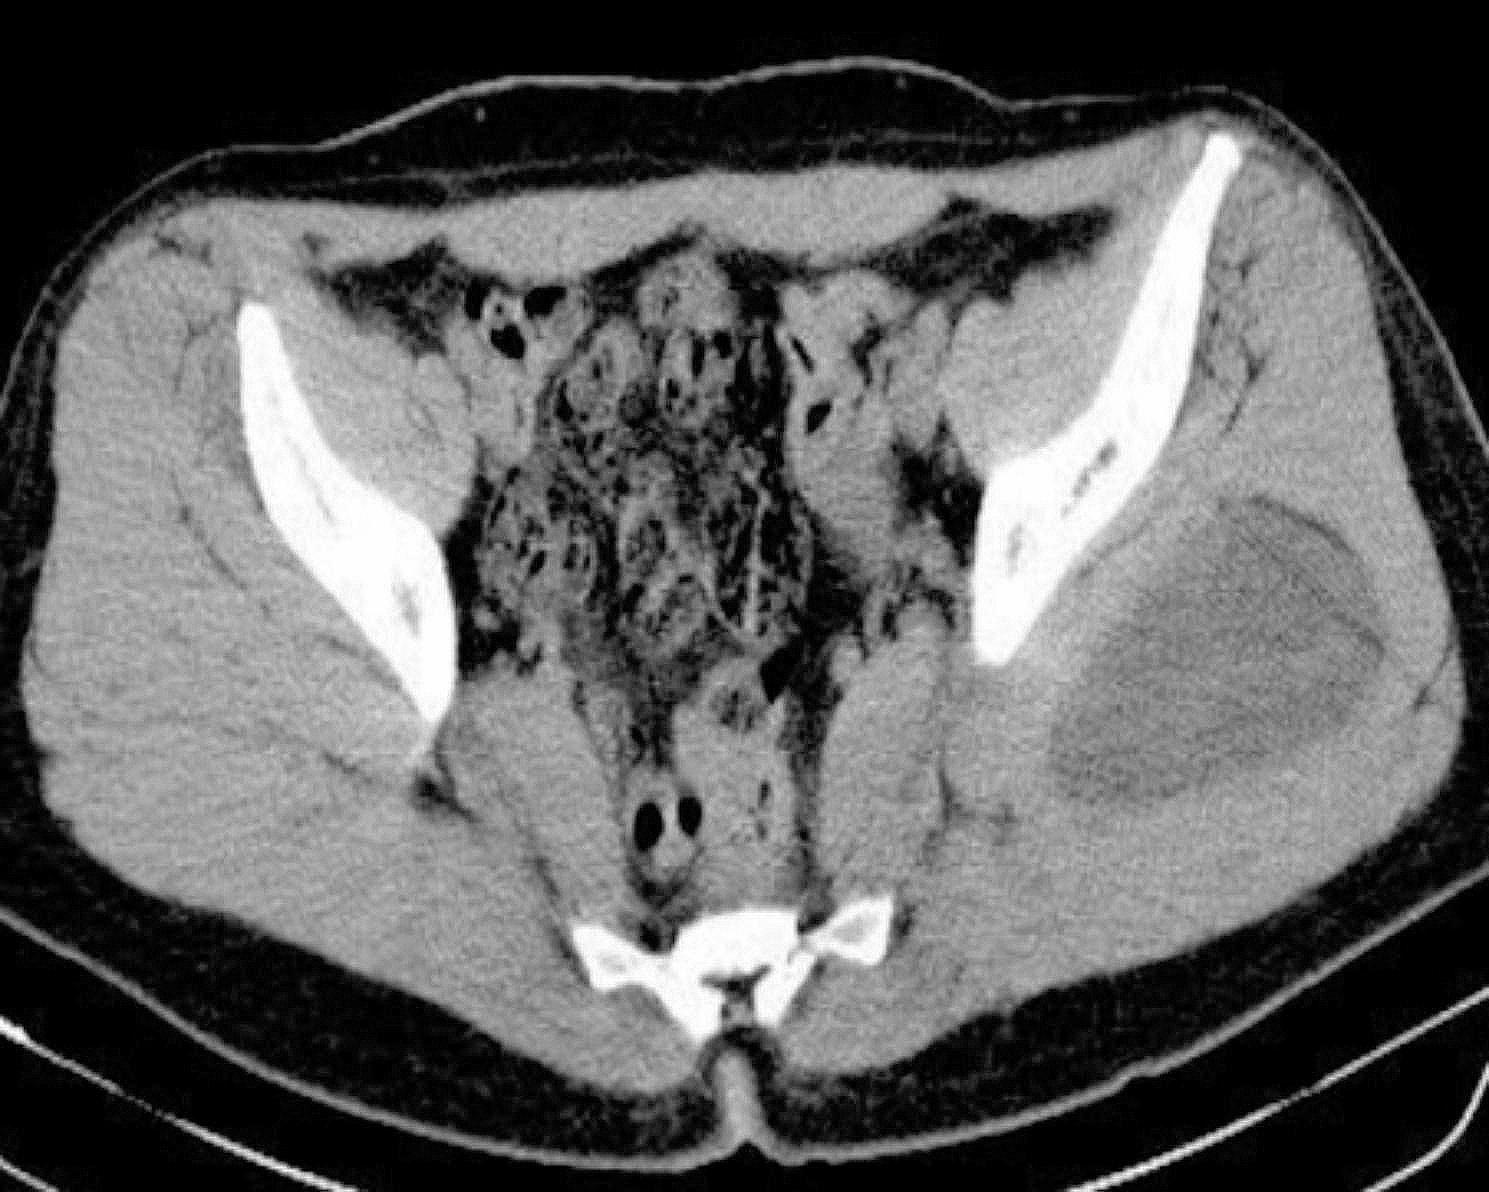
CT scan findings revealed gluteal hydatid cyst with involvement of the iliac bone, the coxo-femoral joint
